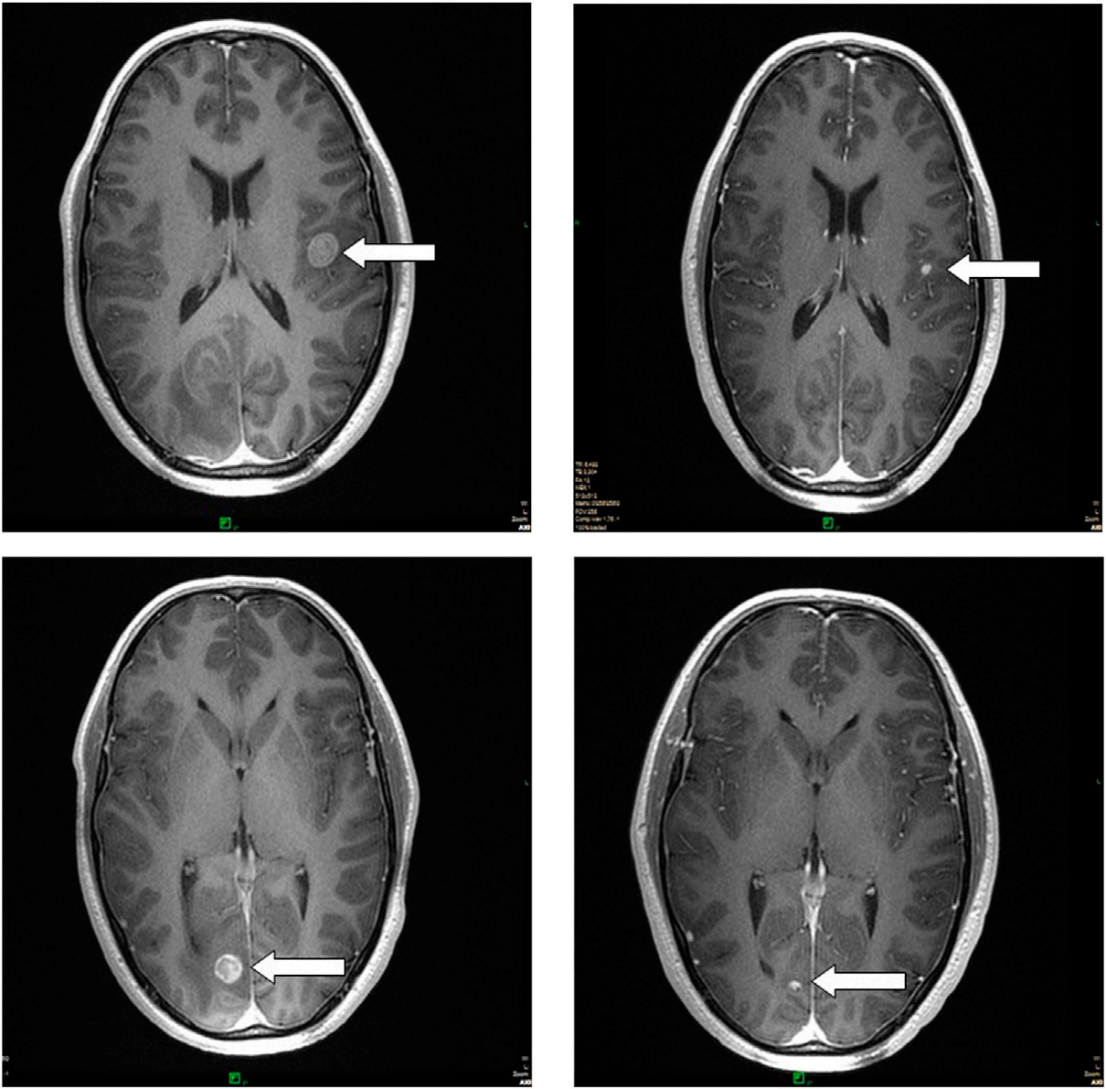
Follow-up MRI scans show complete regression of two brain metastases, 6 months after the radiosurgery, treated simultaneously. The patient received targeted therapy as systemic treatment.
